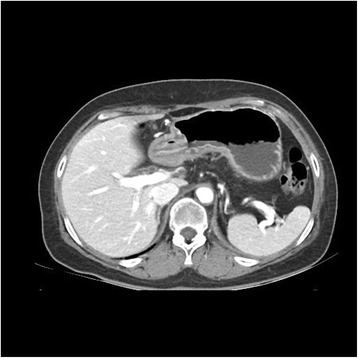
Followed CT scan shows improvement of wall thickening of stomach
